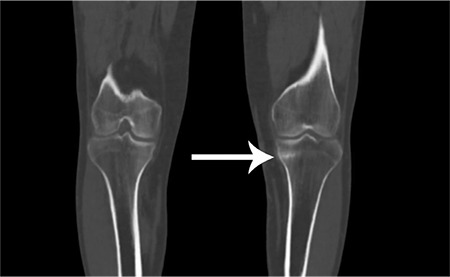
Computed tomography (CT) image from single-photon emission CT (SPECT)/CT fusion study showing a linear fracture in the proximal tibia. While distal and middle third of the tibia are the most frequent sites for stress fractures (almost 50%), stress fractures of the proximal tibia is relatively rare and could be confused with other types of tibial fractures (1). SPECT/CT, which combines the high anatomical resolution of CT with the early-diagnosis capability of SPECT, is an increasingly preferred imaging technique in the diagnosis and follow-up of these patients (2).
